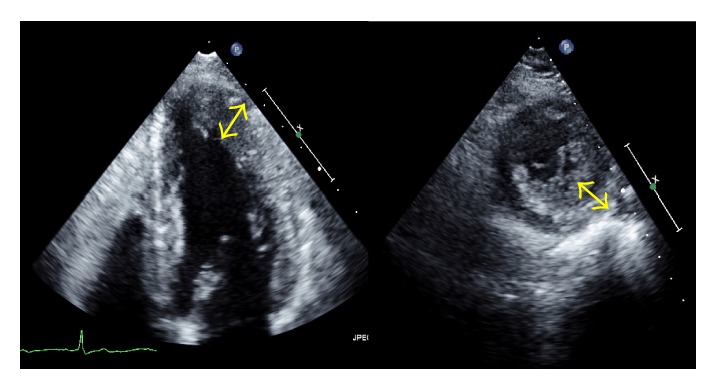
Two-dimensional echocardiography. Hypertrophy of the apical lateral wall was observed in the apical four chamber view (left) and short axis view (right).
